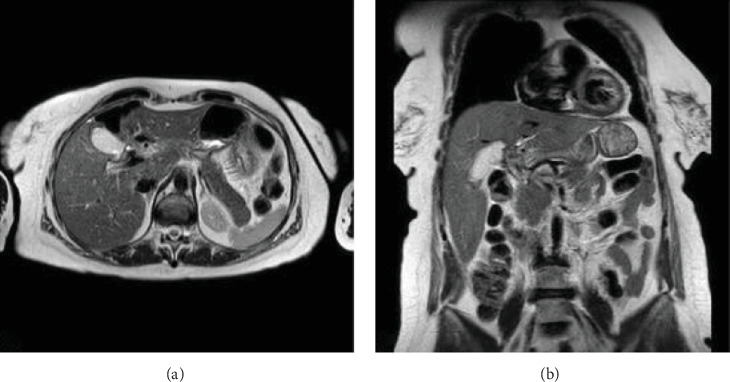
(A) T2 sequence magnetic resonance image in axial projection shows the residual wall of the cystic mucinous neoplasm, (B) T2 sequence magnetic resonance in coronal projection.
